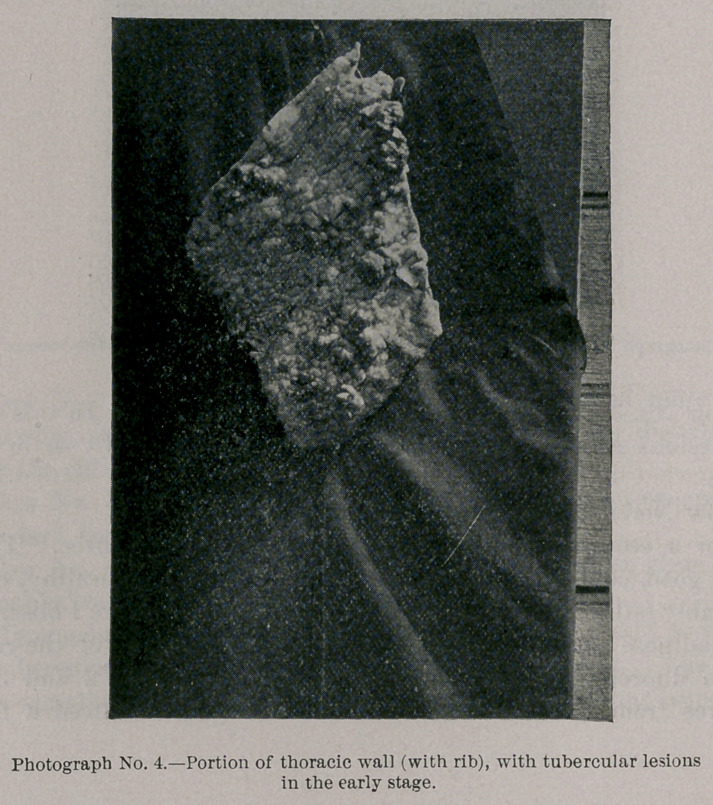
Radiograph No. 4. f8:
